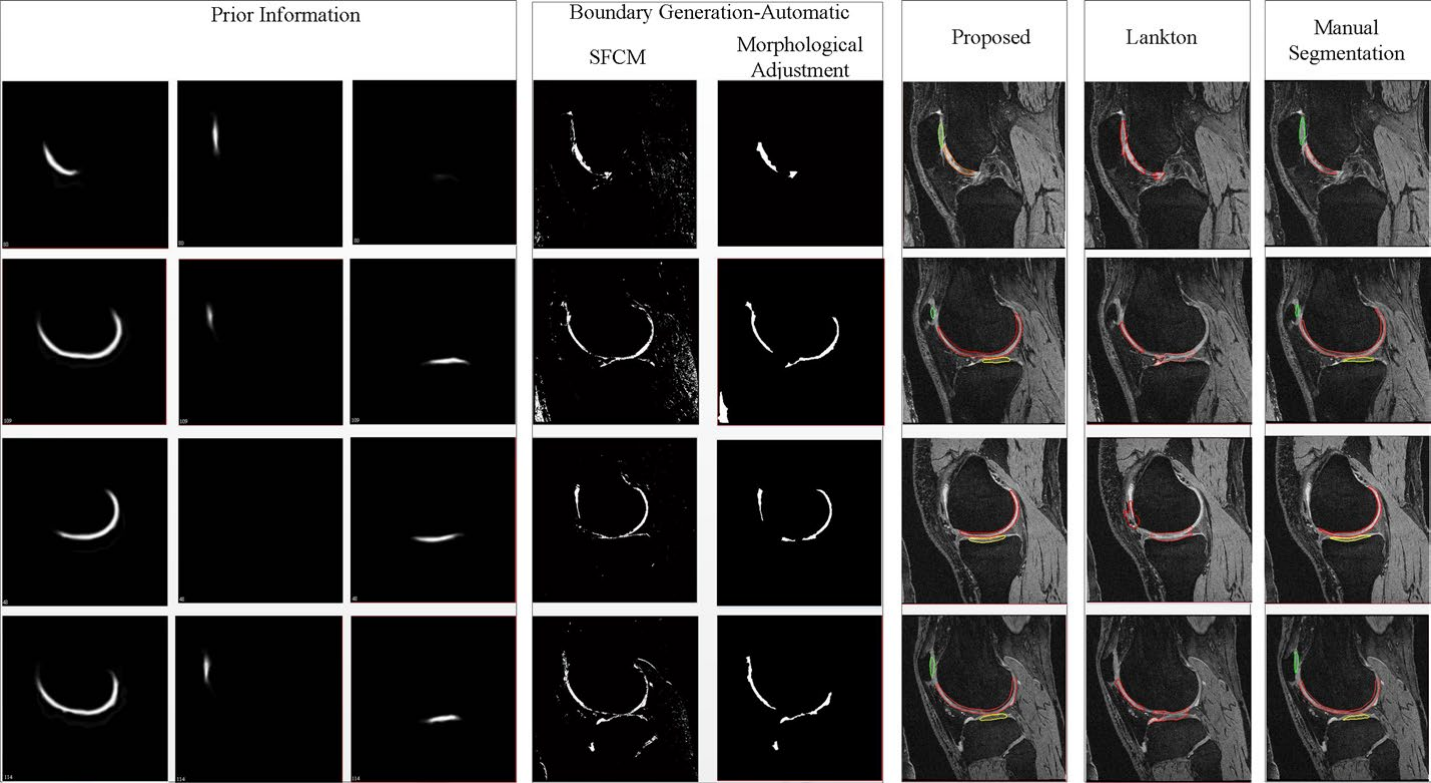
Knee cartilage segmentation for various cases in two dimensions. Each *row* represents a slice of a knee MR image in a single series. a Our template image from normal subjects: femoral (*left*), patellar (*center*), and tibial (*right*) cartilage. b Warping results from the template to the original image: SFCM (*left*) and morphological adjustment (*right*). c The result of Lanktons method. d The result of our proposed method. e The result of manual segmentation for reference
